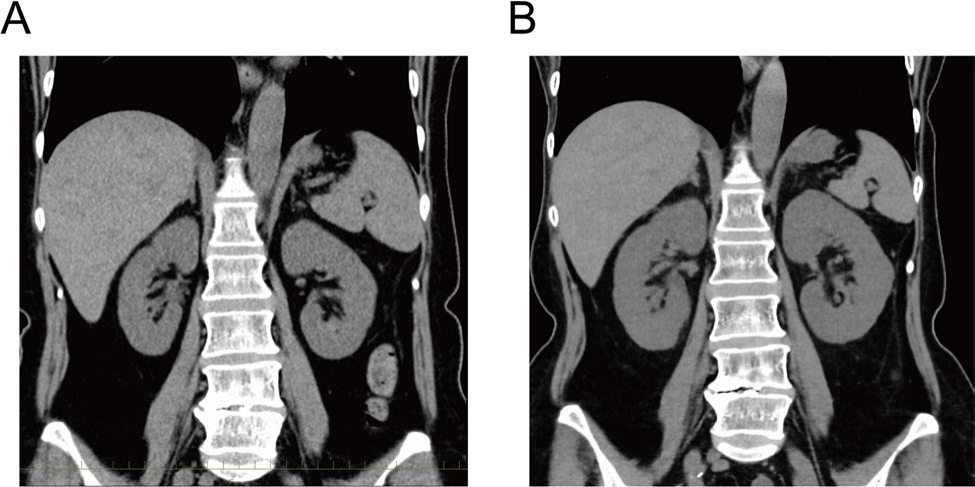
Computed tomography (CT) images of the abdomen. A,  B CT revealed bilateral nephromegaly at the diagnosis (B, right: 11.2 cm × 5.5 cm, left: 10.6 cm× 5.7 cm), compared to 6 years prior (A, right: 9.6 cm × 4.5 cm, left: 9.4 cm× 5.0 cm)
